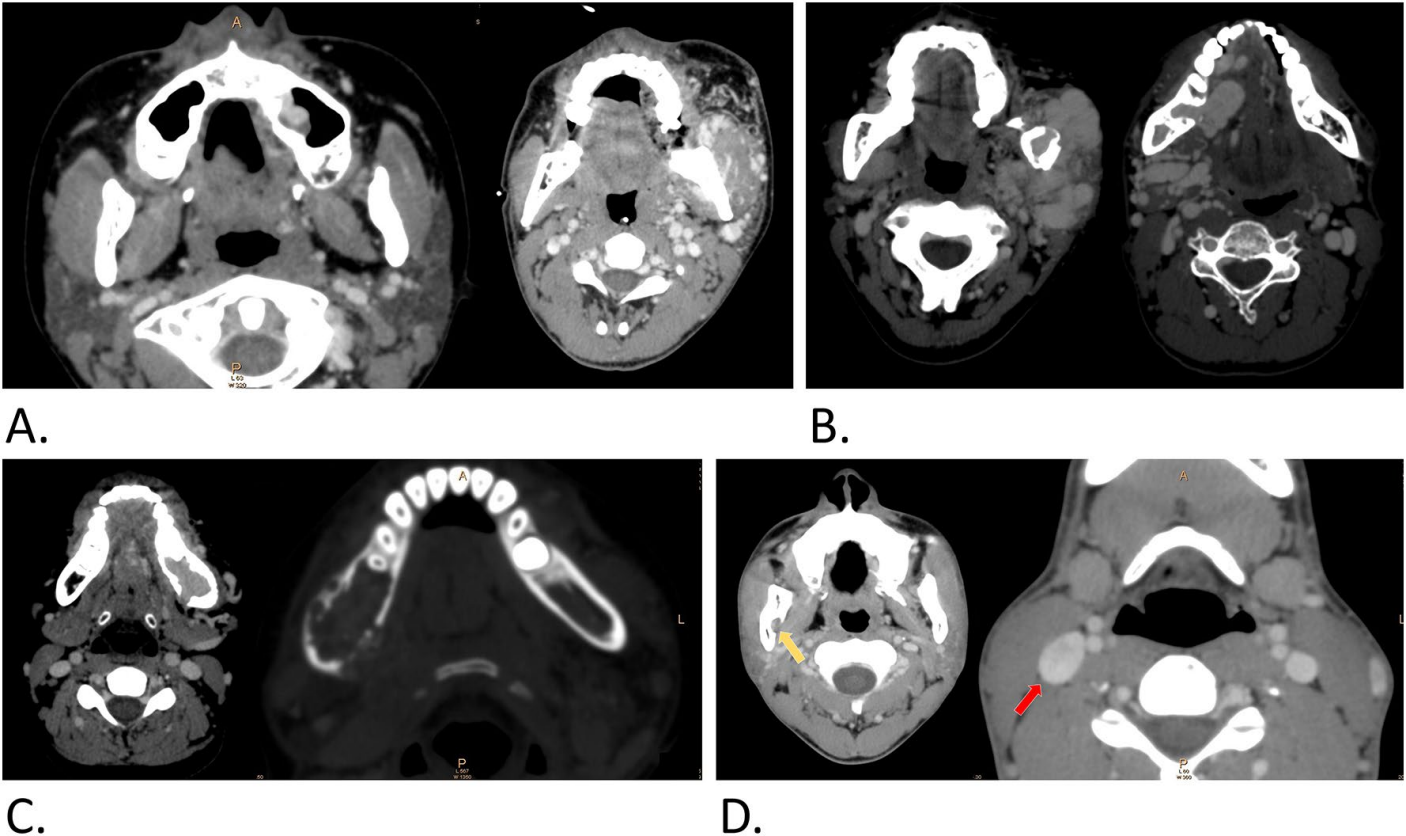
Other manifestations on contrast-enhanced CT of j-AVMs. (a) Involvement of the adjacent maxillary sinus and soft tissue. (b) Enlarged and tortuous draining veins showing abnormal arterial enhancement located inside or adjacent to either side of the jaw. (c) Signs of penetration of large vessels breaking through normally structured cortical bone. (d) Enlarged and wider mandibular foramen (yellow arrow) and enlarged external jugular vein (red arrow) compared with those on the contralateral side.
